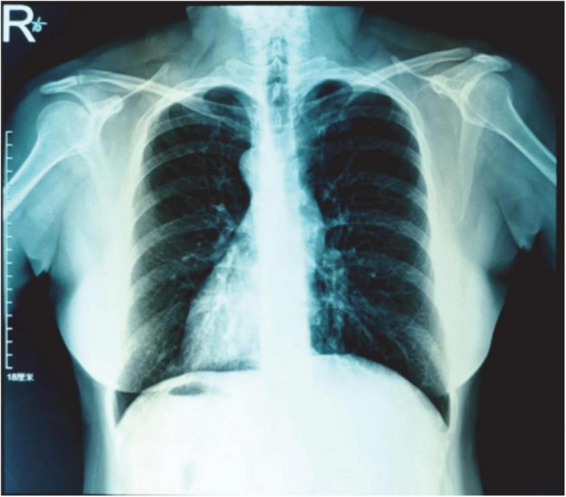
The PA view chest X-ray shows shadows of the heart and aortic arch in the right hemithorax along with gas in the fundus of the stomach on the right side.
